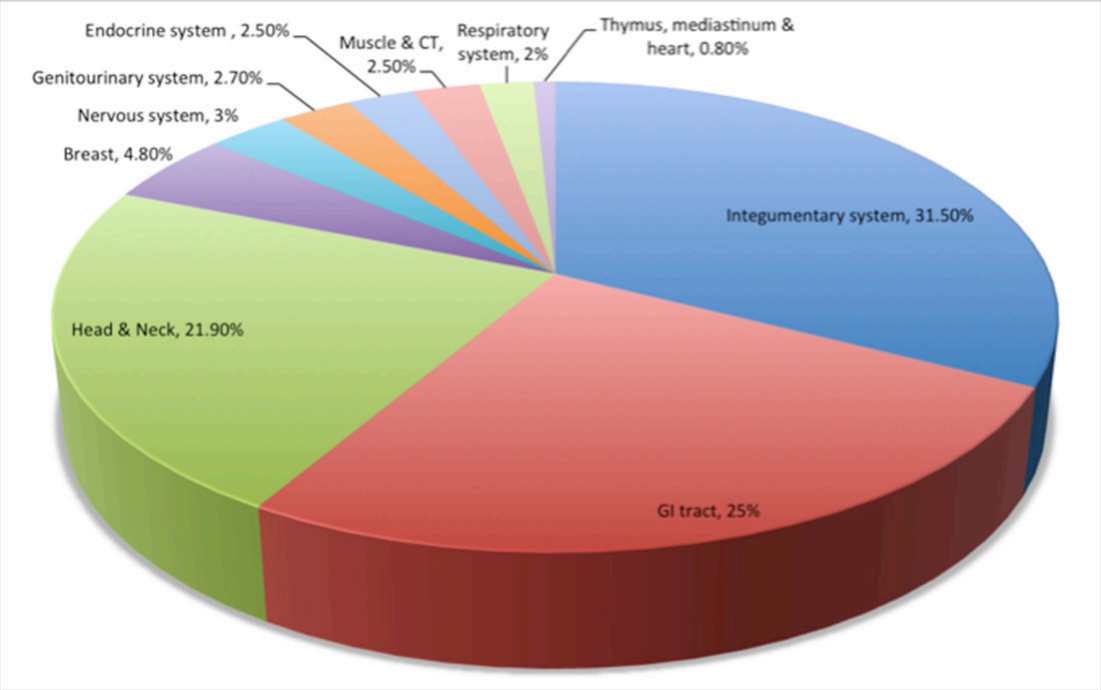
Primary extranodal sites of involvement CT, cardiothoracic; GI: gastrointestinal. *Cases not included here are those that have a known but unclassified primary site and reticuloendothelial system as a primary site.
